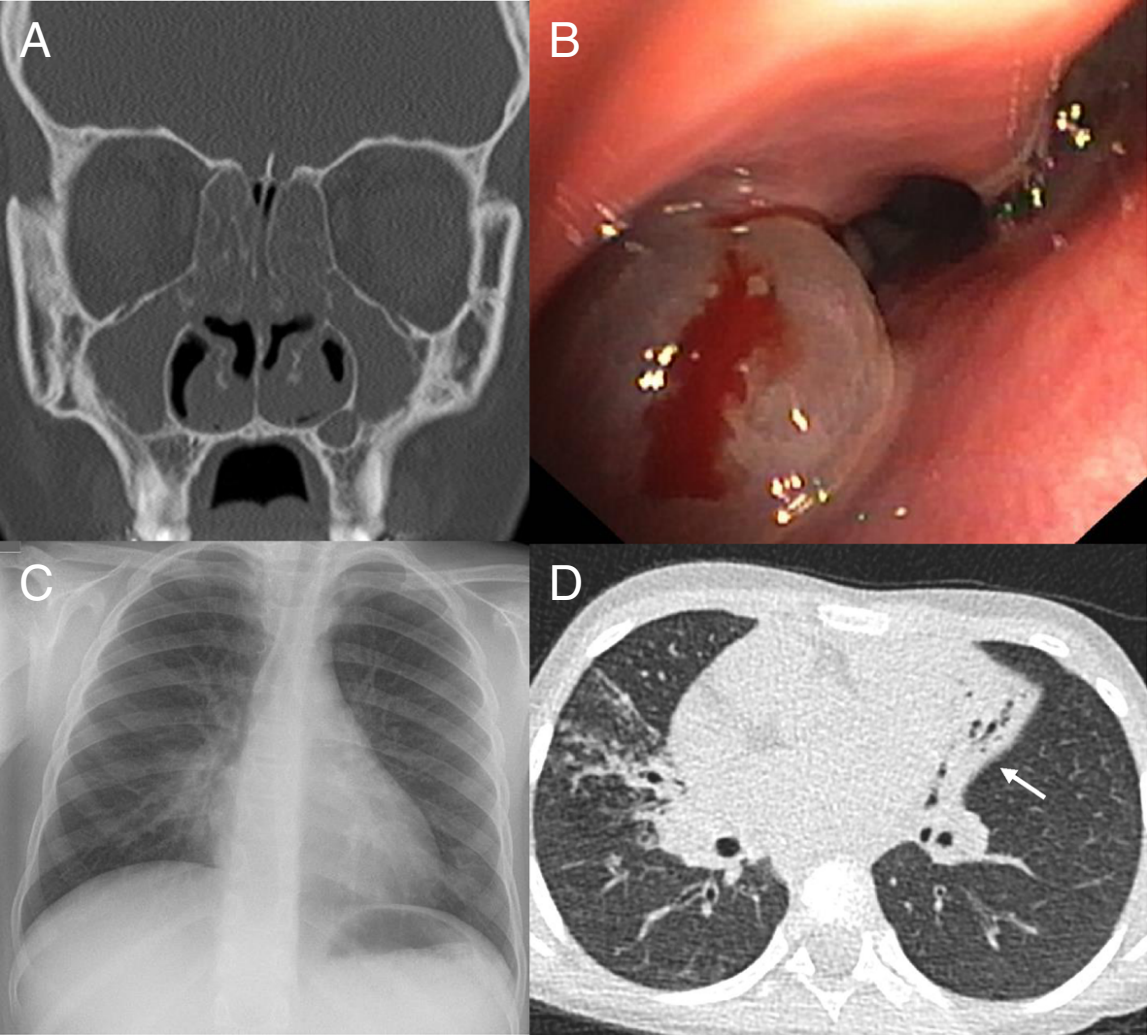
Clinical features of primary ciliary dyskinesia. (A) Coronal computed tomography (CT) scan of a 17-year-old PCD individual showing diffuse pansinusitis with mucosal thickening and polyposis. (B) Endoscopic view showing nasal polyp (same patient). (C) Chest X-ray of a 6-year-old PCD individual with middle lobe atelectasis. Silhouetting of the right heart border is present. (D) Chest CT of a 6-year-old individual with situs inversus totalis. The left-sided middle lobe shows extensive bronchiectasis with volume loss (white arrow). In addition, consolidations and mucous impaction are present in the right upper lobe.
